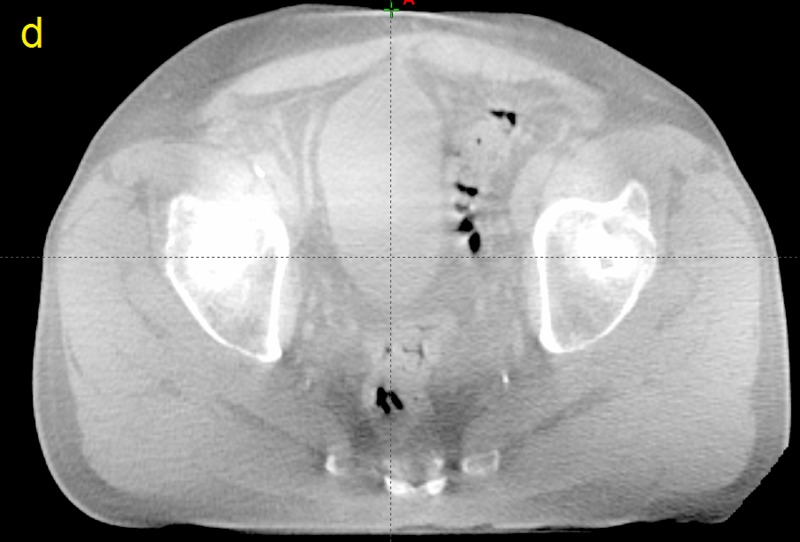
Computed tomographic (CT) image taken from patient P12 d) A slice taken from the cone beam computed tomography of fraction 2 at the same level as the previous images.
